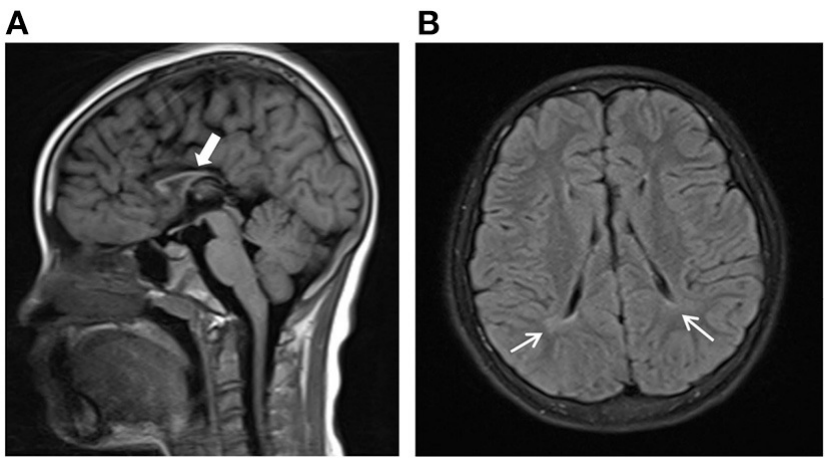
Brain magnetic resonance imaging (MRI) findings of the proband. (A) Dysgenesis of the corpus callosum from sagittal view shown on axial T1-weighted images. (B) Periventricular white matter hyperintense lesions on FLAIR images.
